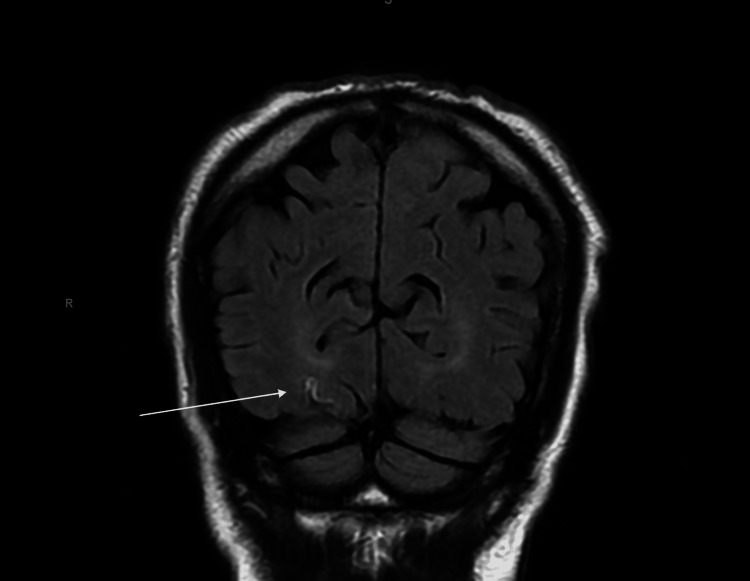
Magnetic resonance imaging without contrast of the brain demonstrated subtle areas of cortically based reduced effusion along the medial aspect of the right occipital lobe, consistent with CJD. White arrow: cortical ribboning sign showing reduced effusion. CJD, Creutzfeldt-Jakob disease
